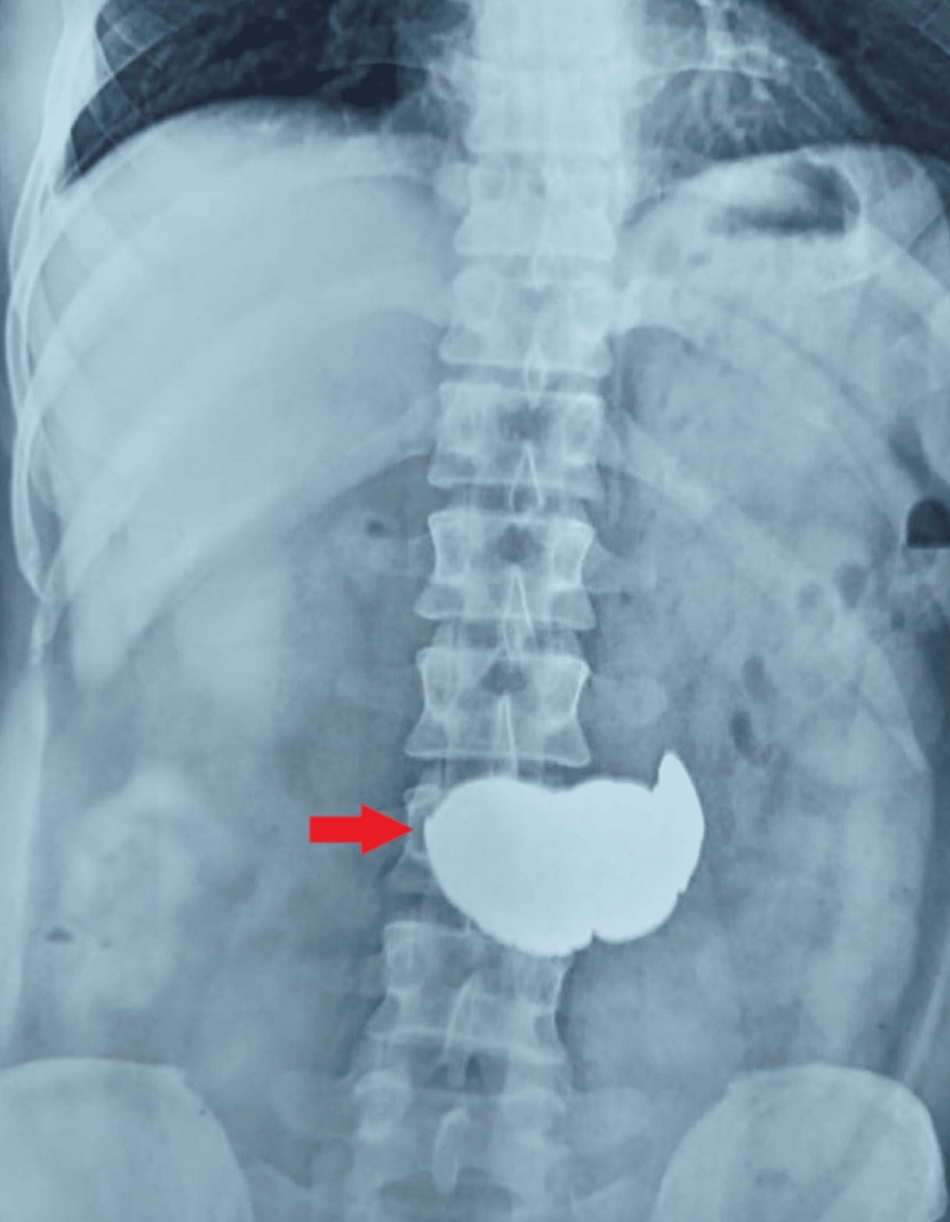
Abdominal X-ray showing heaped-up coins (red arrow) in the stomach
